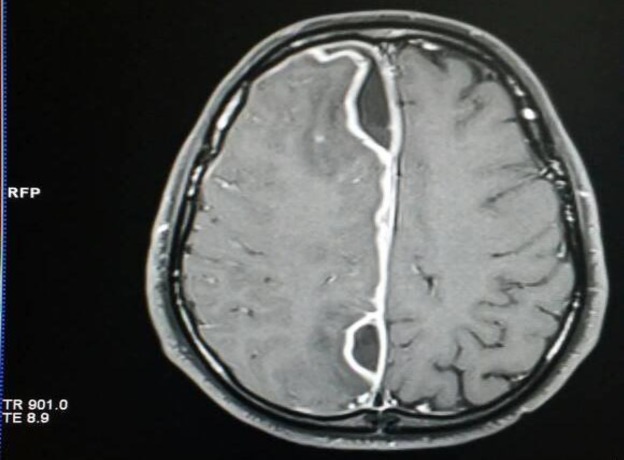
Magnetic resonance image (MRI) of the brain revealing a ring-enhancement lesion in the parasagittal area of right frontal lobe
